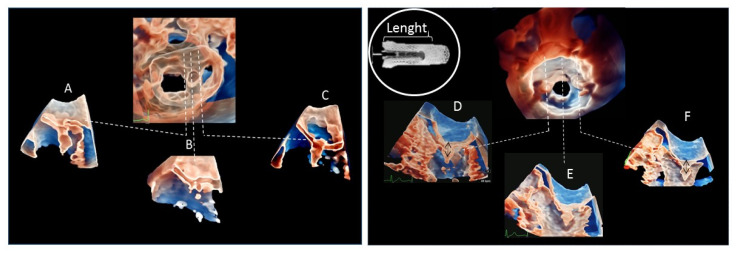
Left Panel: three-dimensional transesophageal (3DTEE) surgical view of a single MitraClip (MC) implantation with a typical optimal final result. From (A–C) consecutive longitudinal 3DTEE sections with transparency rendering from anterolateral to posteromedial. (A): visualization of the MC and the grasped anterior leaflet. (B: both leaflets are well visualized and the inserted leaflet portion may be measured. (C): MC depth is well defined. Right panel: 3DTEE reconstruction with transparency rendering of the mitral valve from left ventricular view in an atypical case with the insertion of 2 MC resulting in a single central orifice. From (D–F) consecutive longitudinal sections from anterolateral to posteromedial. (D,F) longitudinal sections of the 2 MC implanted in lateral (D) and medial (F) position, respectively. (E): longitudinal section of the central residual mitral orifice. White encircle panel: MC length that has been compared to the 3D images. Black arrows showing the MC length measurements corresponding with the nominal dimensions.
